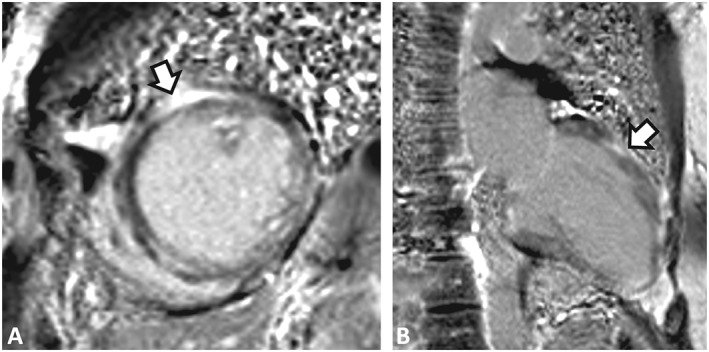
Cardiac magnetic resonance imaging. (A) Mid short axis late gadolinium enhancement (LGE) image. (B) Two‐chamber LGE image. There is a distinctive pattern of diffusely located “patchy” transmural delayed hyper‐enhancement compatible with infiltrative cardiomyopathy and fibrosis of the left ventricle. This pattern is not indicative of ischaemic cardiomyopathy. There is subepicardial LGE along the mid‐anterior wall, which can be seen in myocarditis.
